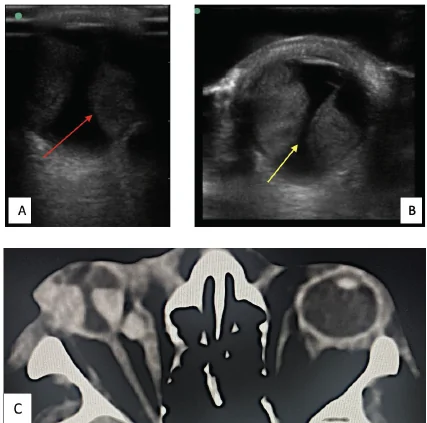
Case 5. A patient with right eye choroidal detachment: A) conventional method of ocular ultrasound (red arrow); B) modified water bath technique (yellow arrow); and C) computed tomography image of the globe injury.
